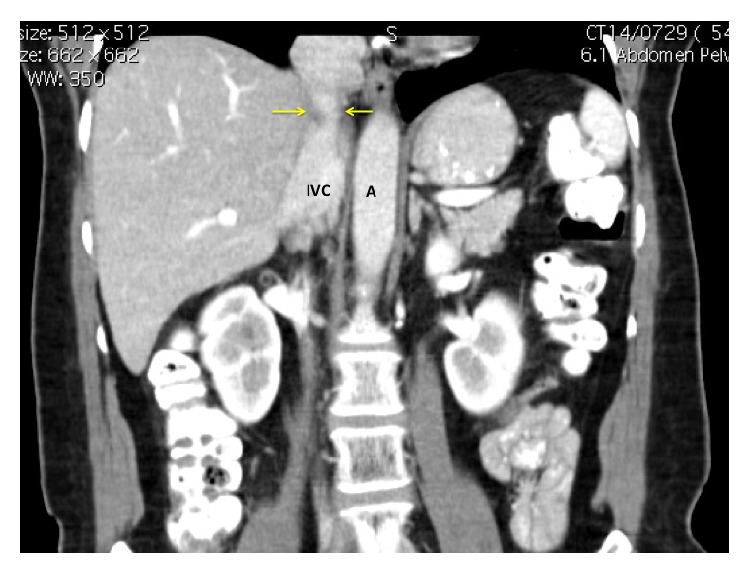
Coronal CT images marked with yellow arrows that point to the area of involvement of the retrohepatic IVC at the superior border of the liver. This area corresponds to the metastatic deposit on the axial images in Figure 1.
